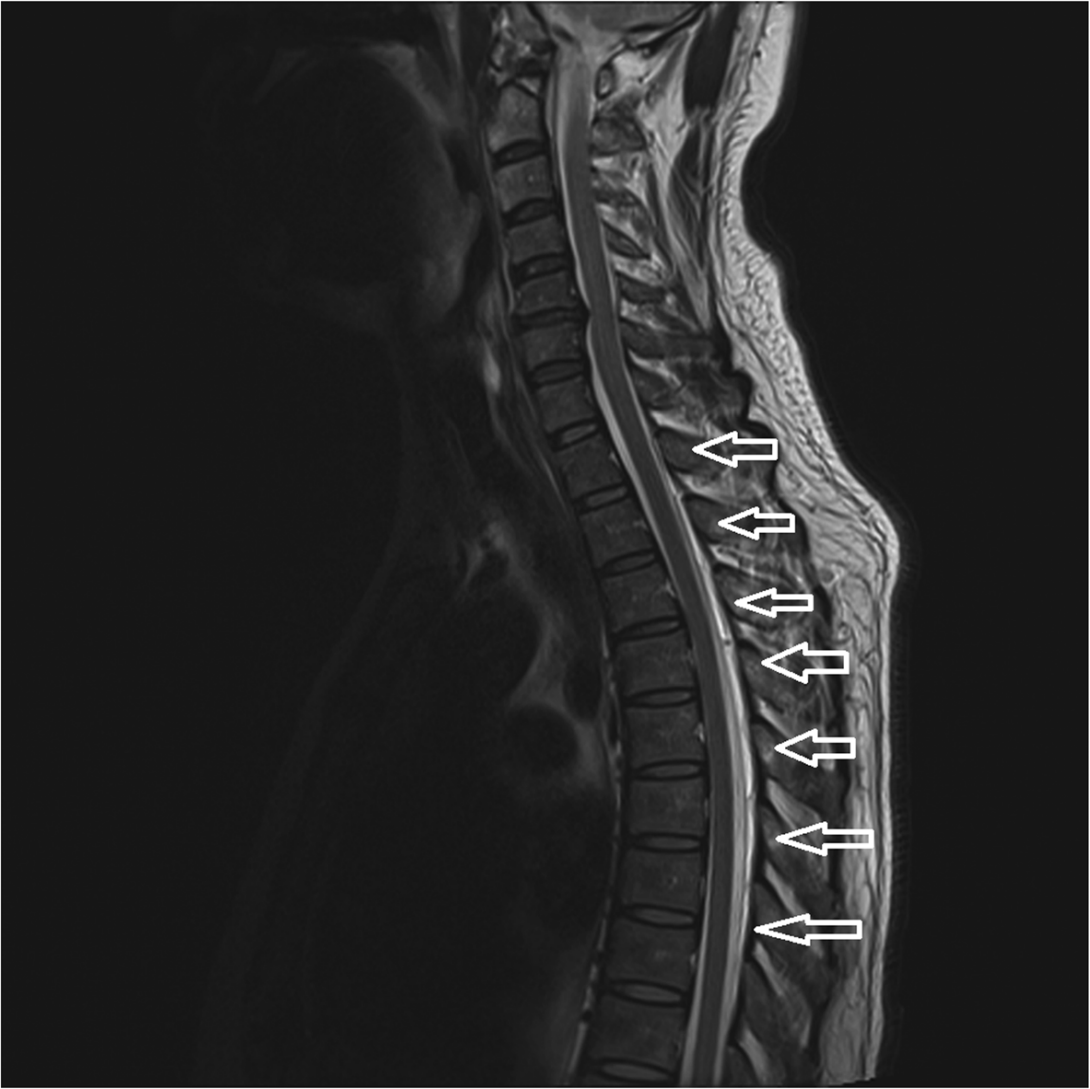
T2-weighed MR image revealed epidural fluid accumulation at the dorsal aspect of the T1–9 spinal canal. Arrow: epidural fluid accumulation
